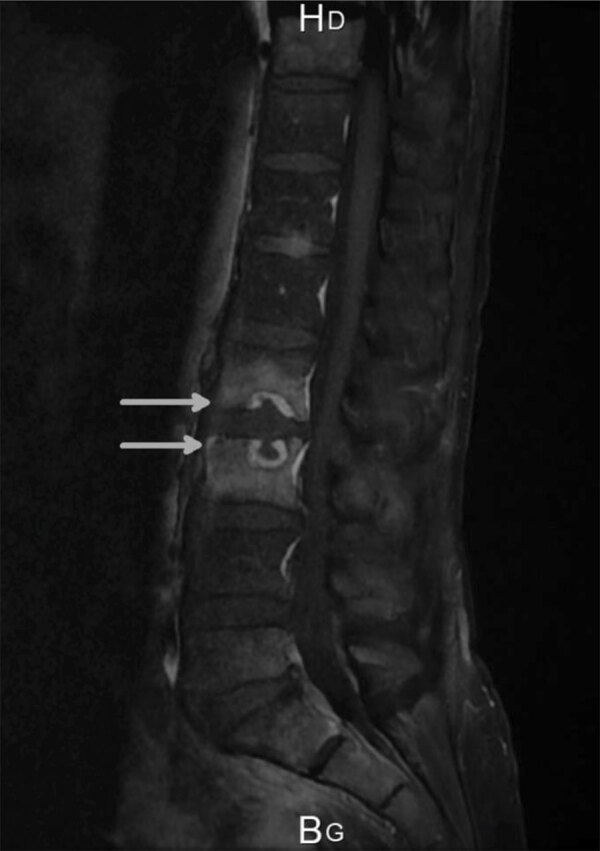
Sagittal gadolinium magnetic resonance image of spine of a patient in France with spondylodiscitis caused by *Nocardia neocaledoniensis*. Image shows erosion of the cortical end plate of L2 and L3 (arrows).
